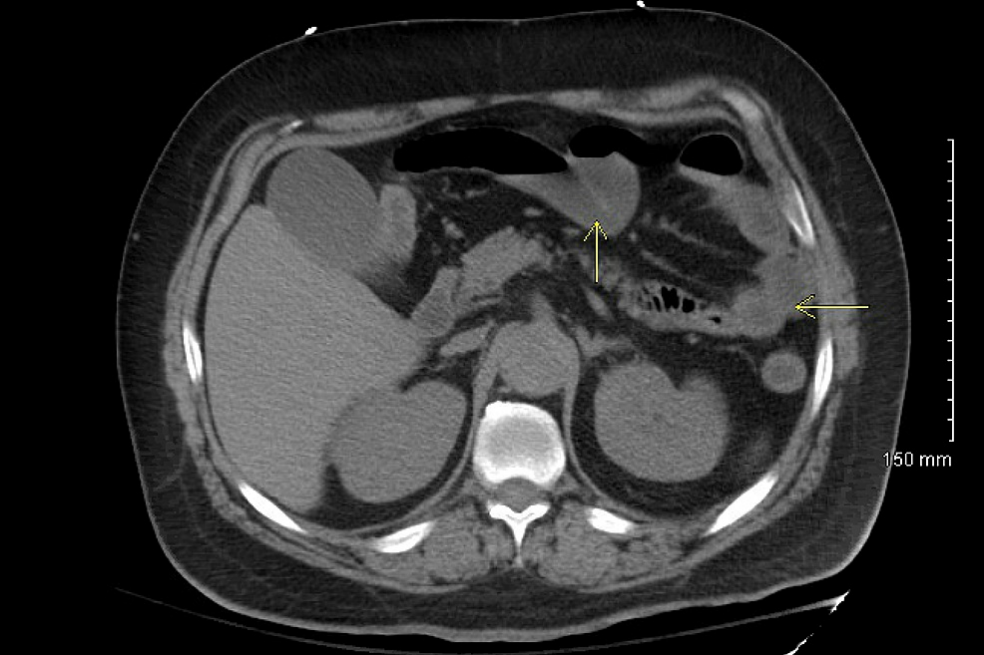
Computed tomography (CT) scan of the abdomen without contrast showing mild nonspecific fluid distension of small bowel and portions of colon consistent with enterocolitis
